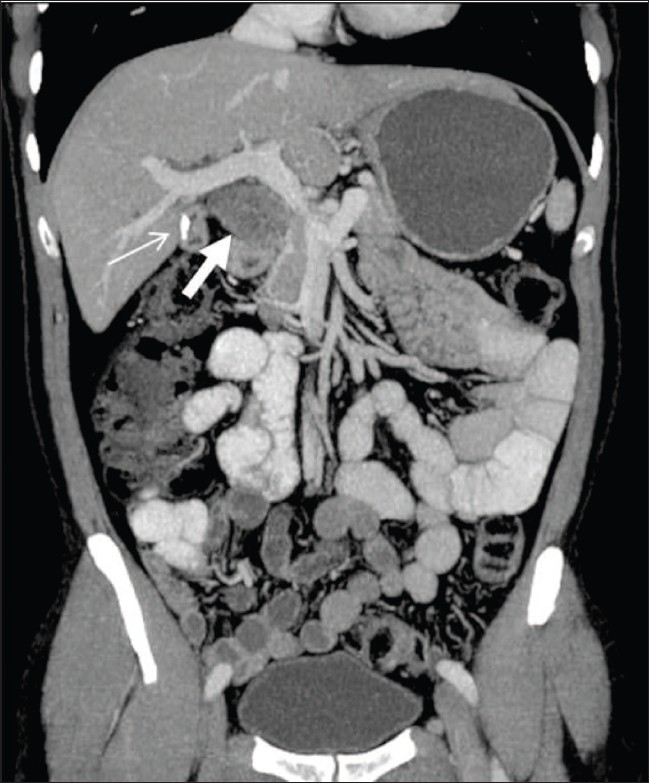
Coronal reformatted CT scan reveals a lesion (thick white arrow) in the porta hepatis extending caudally till the level of the duodenum with dilated intrahepatic biliary radicles. Also seen is a gallstone within a partially distended gallbladder (thin white arrow)
